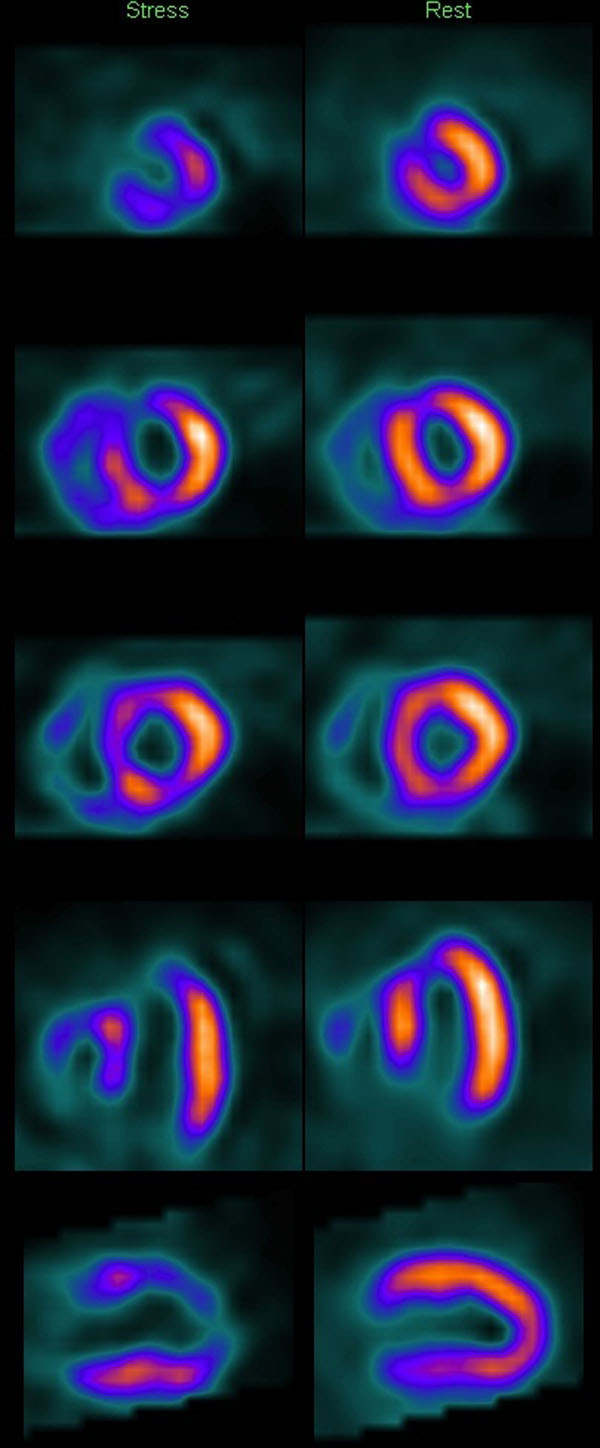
SPECT image demonstrating reduced tracer uptake in the anterior and inferior walls at stress. Note extension into the apex which is also apparent with whole heart 3D CMR perfusion.
